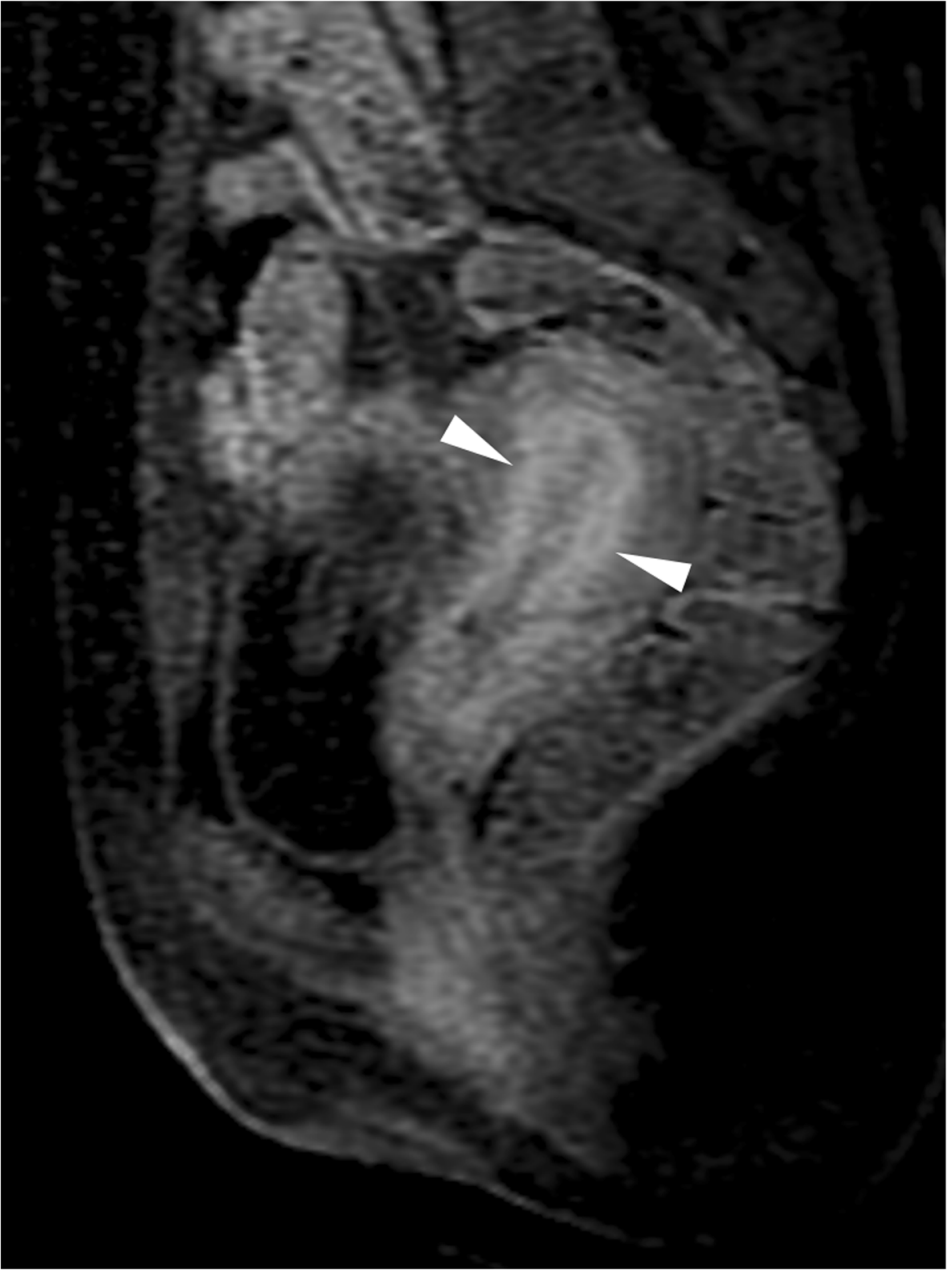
MRI appearance of endometritis in a 38-year-old woman with pelvic pain. Sagittal gadolinium-enhanced fat-suppressed T1-weighted image shows contrast enhancement of the endometrium and inner myometrial layer (arrowheads)
